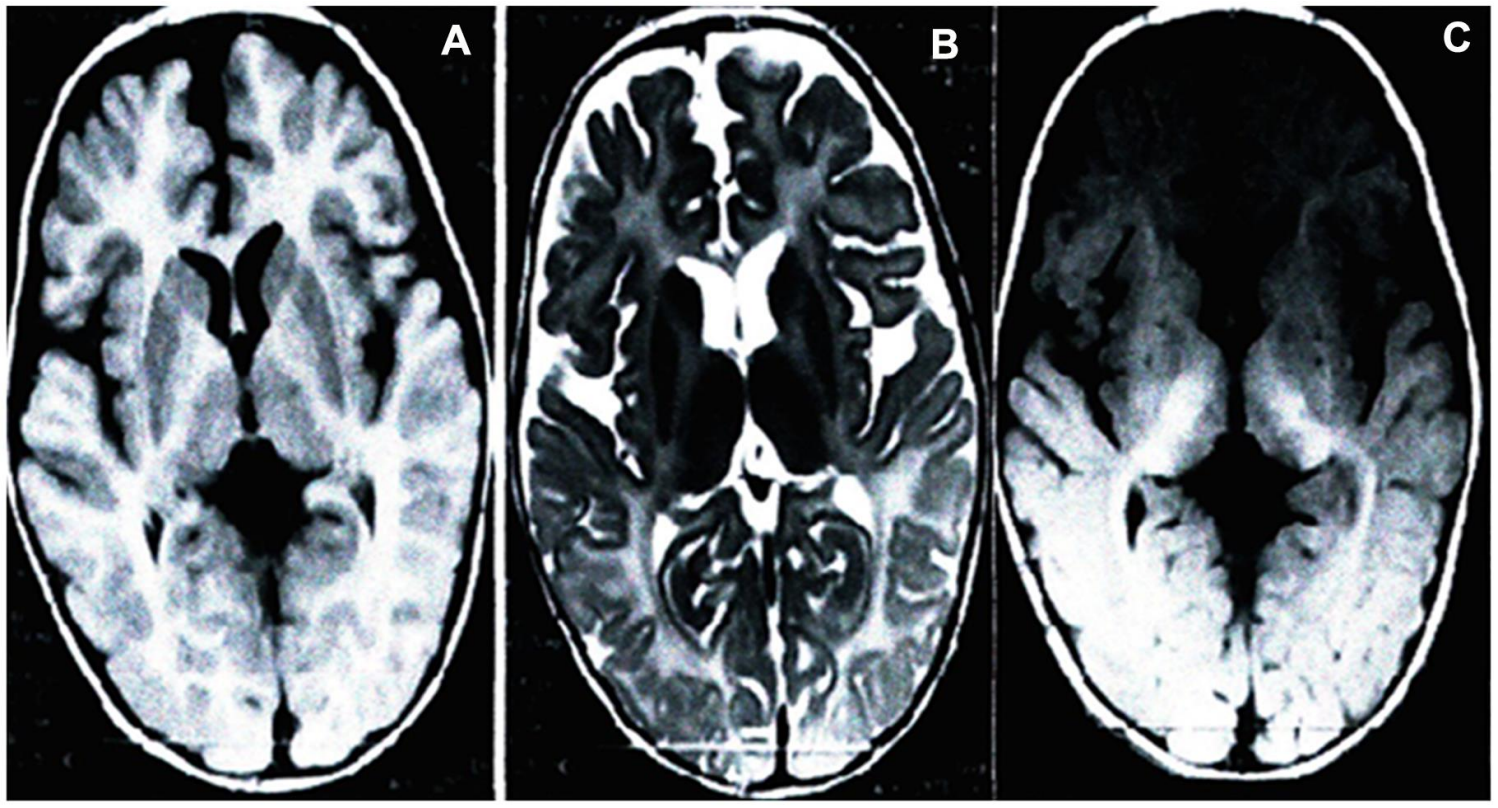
Brain MRI of the proband. Scans showed significantly development delay of white matter, poor myelin formation, close to the level of myelin development in neonates, and reduced volume of white matter throughout the brain. Axial T1-weighted (A), T2-weighted (B) and FLAIR (C).
